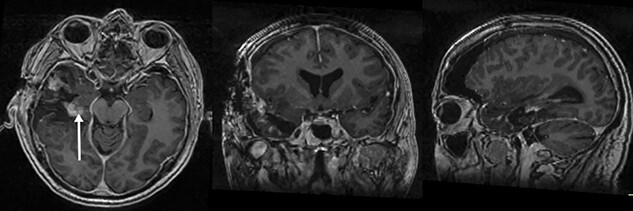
(A–C) Post-operative contrast T1W MRI (axial, coronal and sagittal) showing significant lesional reduction but medial lesional enhancement (arrow) representing a STR.
